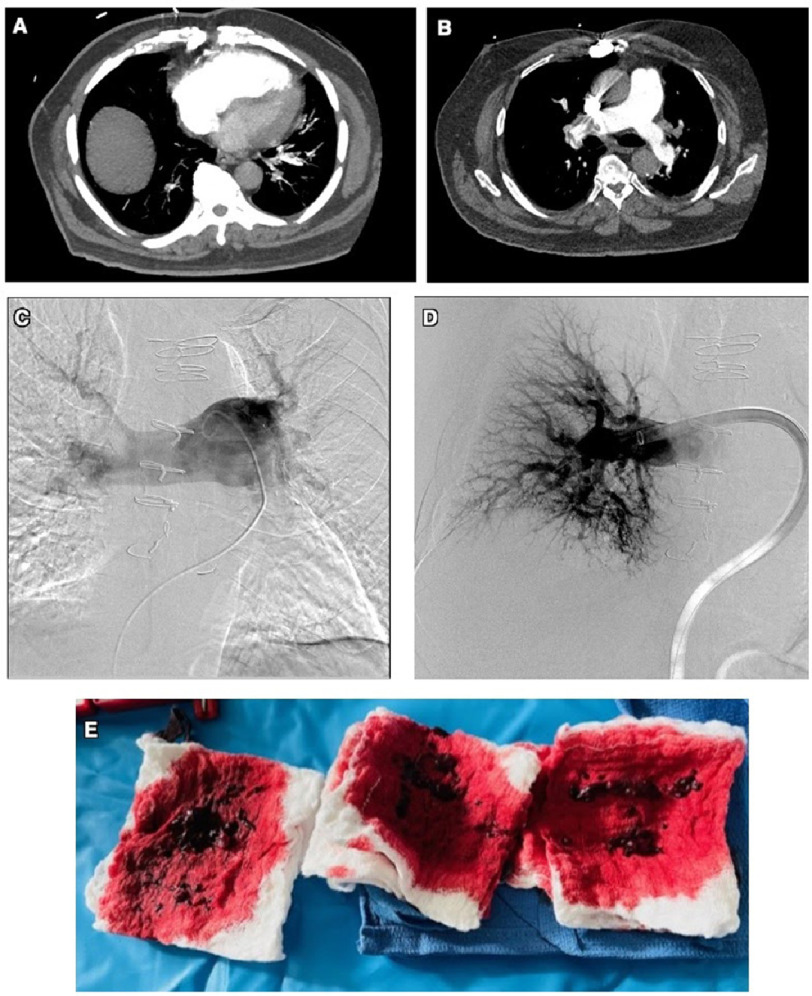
Computed tomography angiogram of the chest showing extensive bilateral pulmonary embolism with near occlusive thrombus in the distal right pulmonary artery and non-occlusive thrombus in the left lower lobe and upper lobe pulmonary arteries (A). Axial cardiac view showing an enlarged right ventricle with a right ventricle/left ventricle ratio of 1.68:1, indicating right heart strain (B). Angiogram showing a near-occlusive thrombus in the distal right pulmonary artery (C). Post aspiration angiogram showing restored flow (D). A large amount of clot was extracted, with no haemodynamic instability (E).
